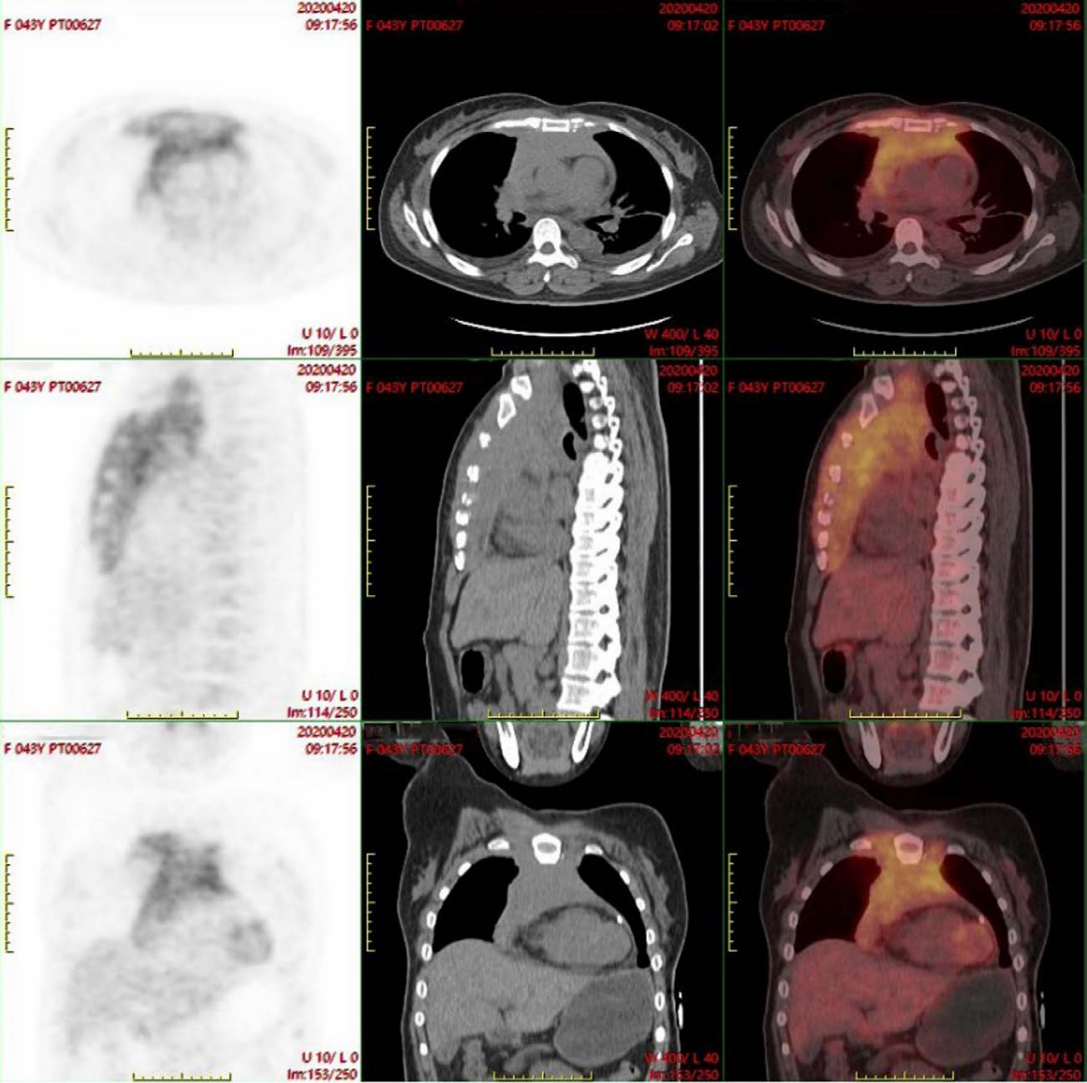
Images from the pectoral PET/CT. Irregular stripe-like softtissue thickening is seen in the anterior mediastinum, mostly located in the anterior superior mediastinum, with diffuse and heterogeneous increased fluorodeoxyglucose uptake, the size is about 8.4 cm × 3.2 cm × 16.3 cm, and the maximum standard uptake value is about 5.8. PET/CT = positron emission tomography/computed tomography.
